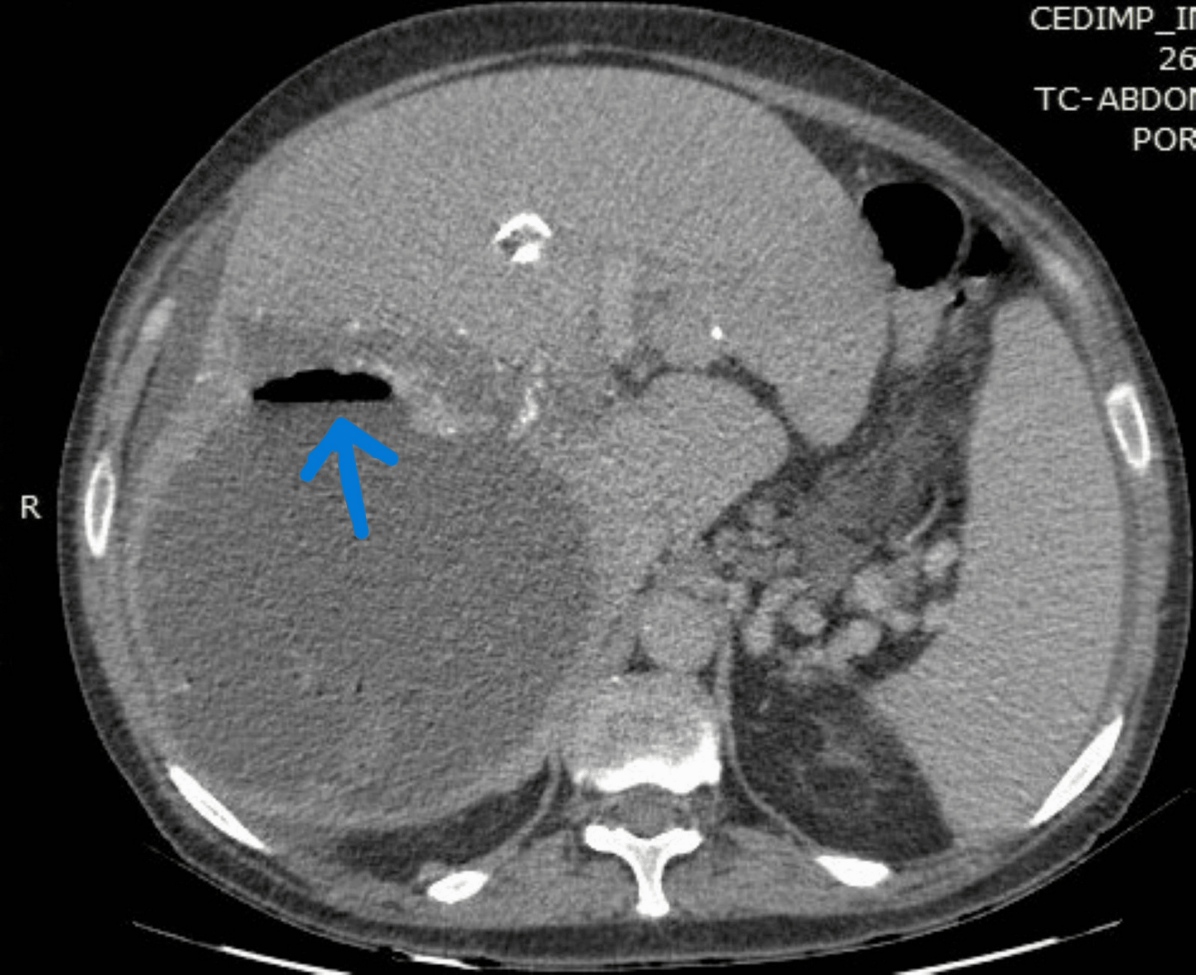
Computed tomography scan during hospitalization showing right lobe air-fluid level (blue arrow).
